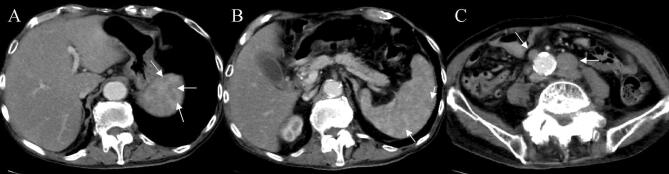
A CT examination revealed no distinct nodules or masses in the liver, but mild splenomegaly, multiple hypodense splenic lesions (a, b), and para-aortic lymph-node swelling (c)
